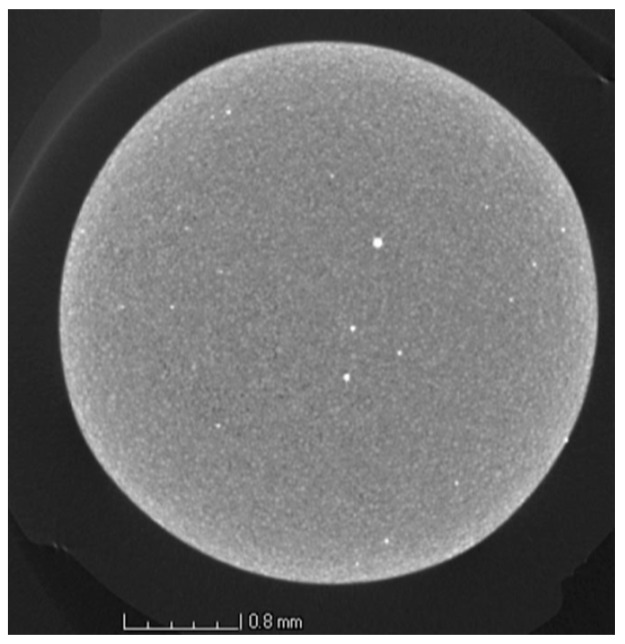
X-ray CT slice image of the powder from Batch 1, showing high density inclusions, visible as bright particles.
